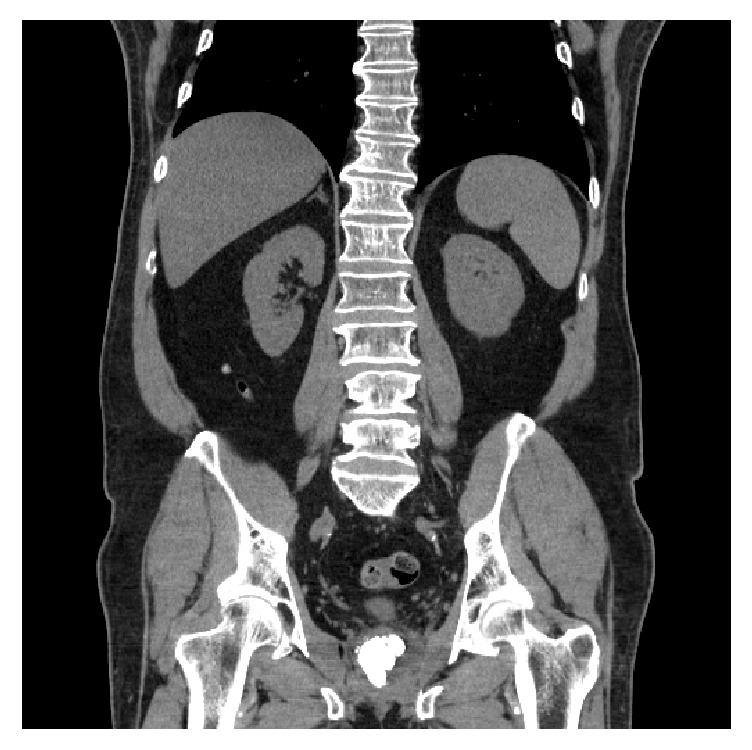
Coronal view of CT abdomen and pelvis noting a large burden of prostate stones.
